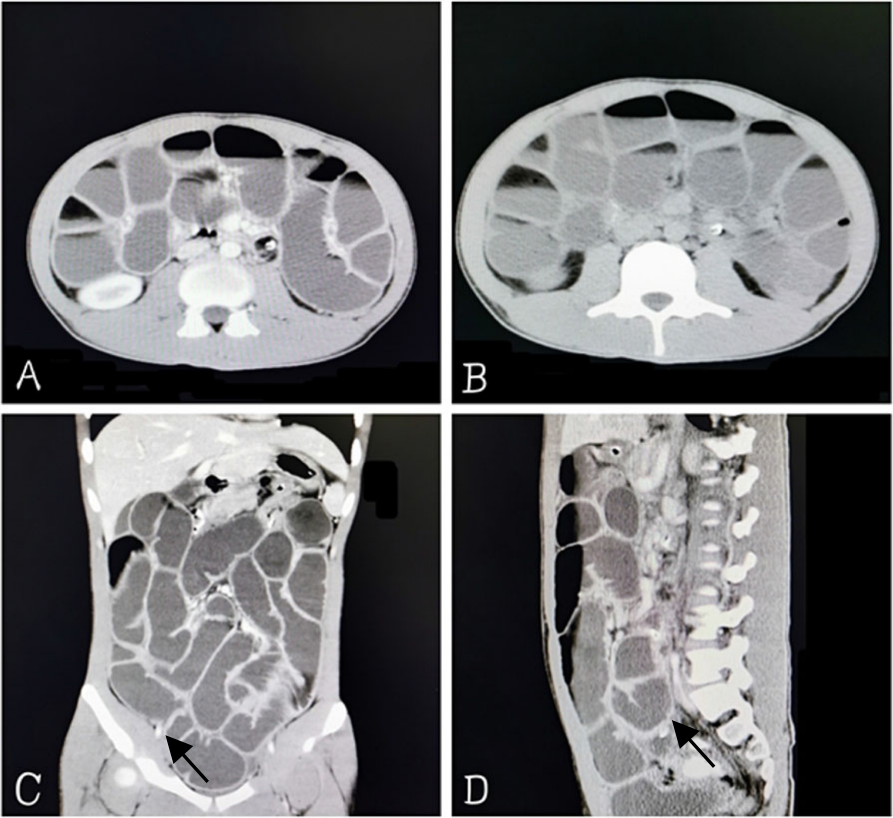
Images from abdomen CT. Panels a and b: CT of the abdominal transverse plane. Intestinal dilation, gas and fluid can be seen in the intestine. Panel c: CT of the anterior abdomen, sagittal. A cystic membrane is visible around the intestine, and the intestinal wall is abnormally thickened (shown with arrow). Panel d: CT of the abdominal sagittal plane. A fibrous membrane is visible around the intestine (shown with arrow)
